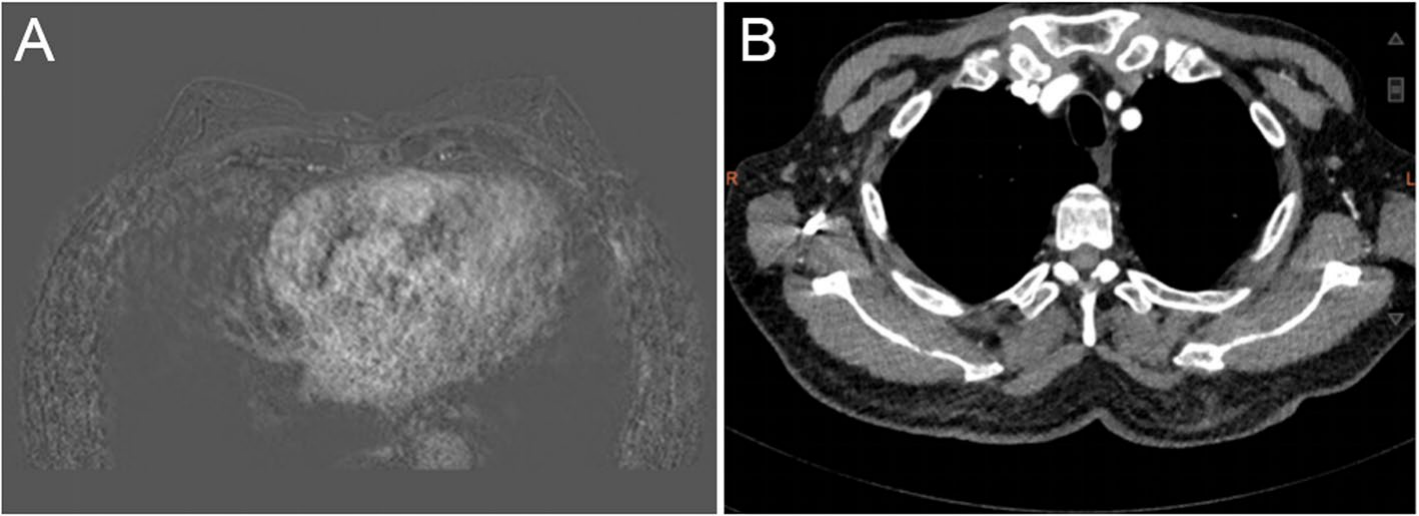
Imaging examination findings of a patient with accessory breast cancer. A Breast MRI showed no abnormal mass. B CT showed no enlarged lymph nodes in the right armpit
